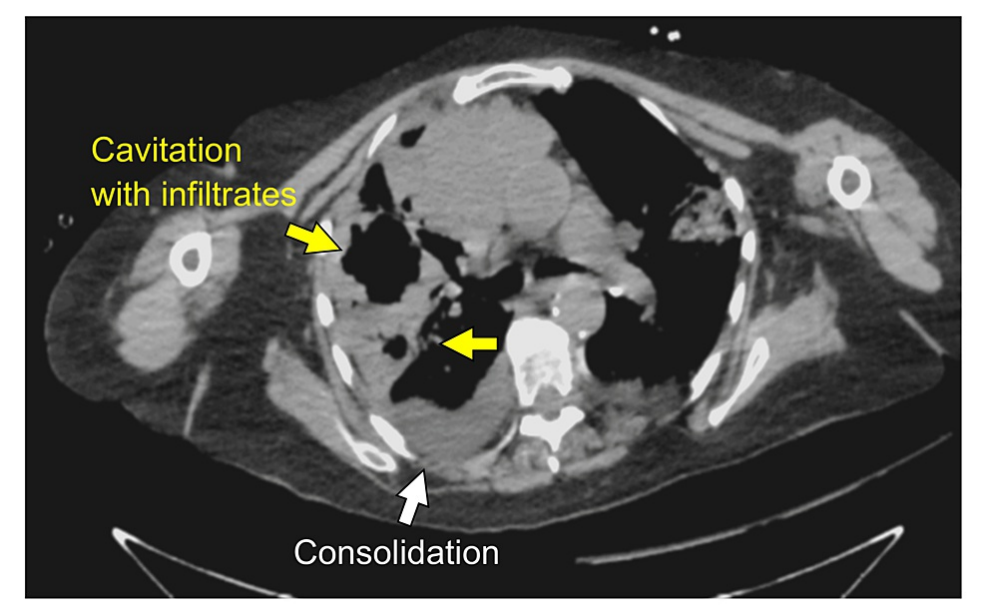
CT scan of the patient's thorax. CT scan of the patient's thorax showed cavitation in the right lung, which increased in volume. Other findings include bibasilar atelectasis, consolidation, ground-glass opacification, and infiltrates.
